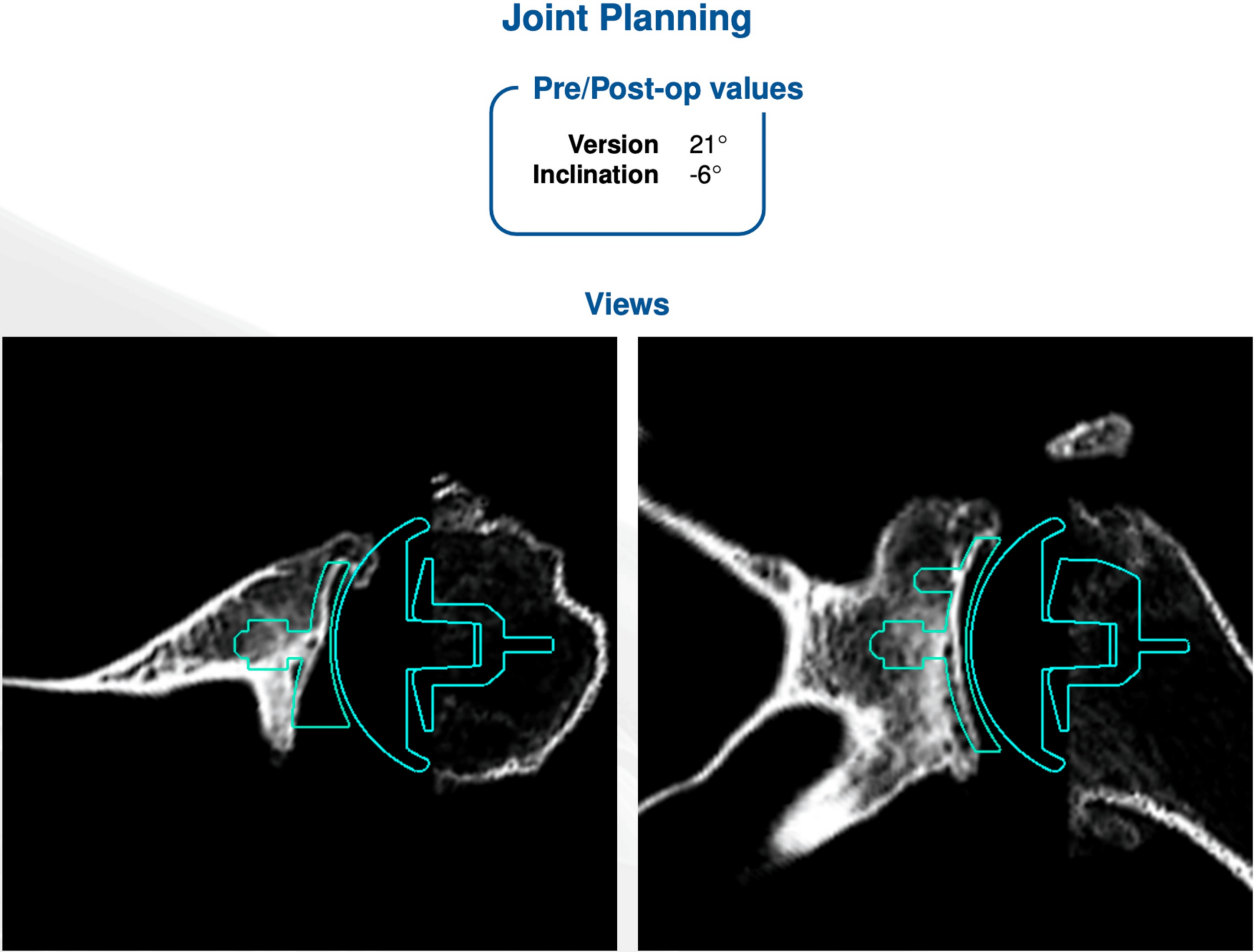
Example of CT scan planning prior to aTSA based on the patient’s preoperative version. A posterior wedge polyethylene implant had been chosen. aTSA, anatomic total shoulder arthroplasty.
